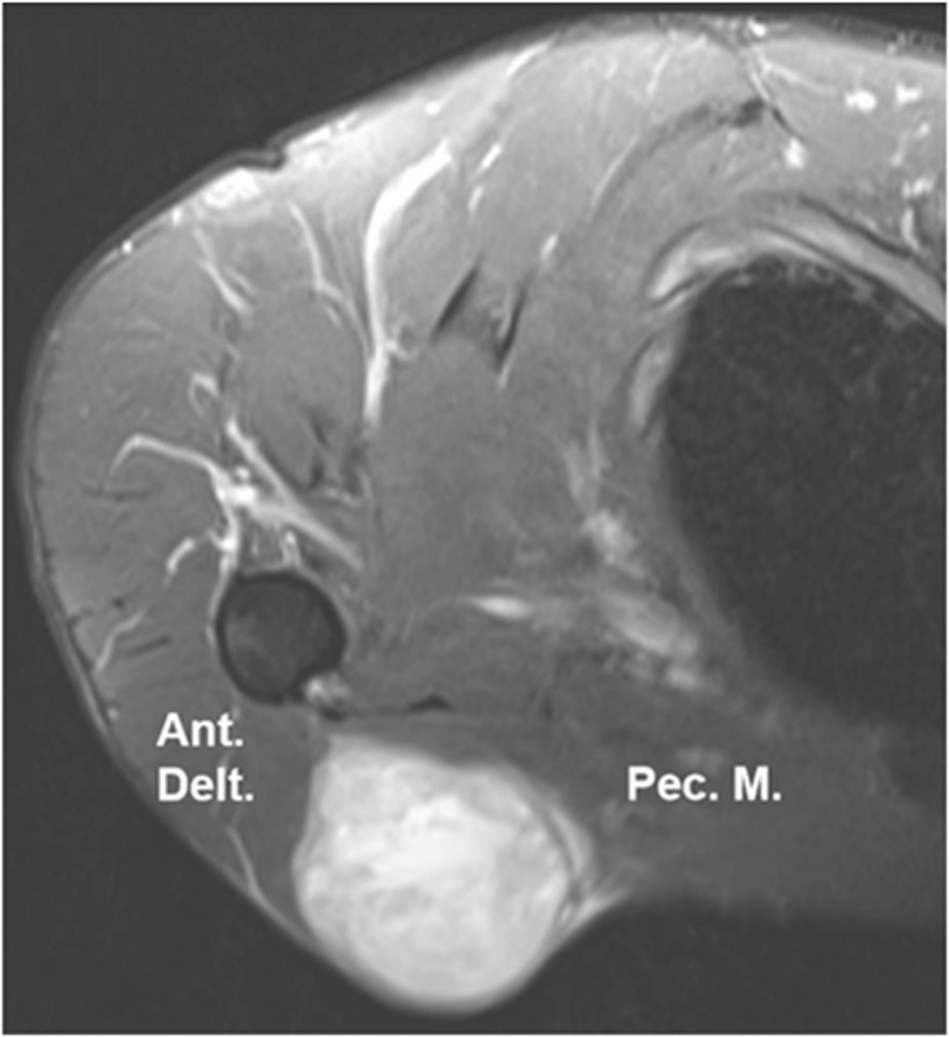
T2 MRI sequence in the axial plane that demonstrates the involvement of anterior deltoid (Ant. Delt.) and pectoralis major (Pec. M.) muscles by this patient’s soft tissue sarcoma pre-operatively
